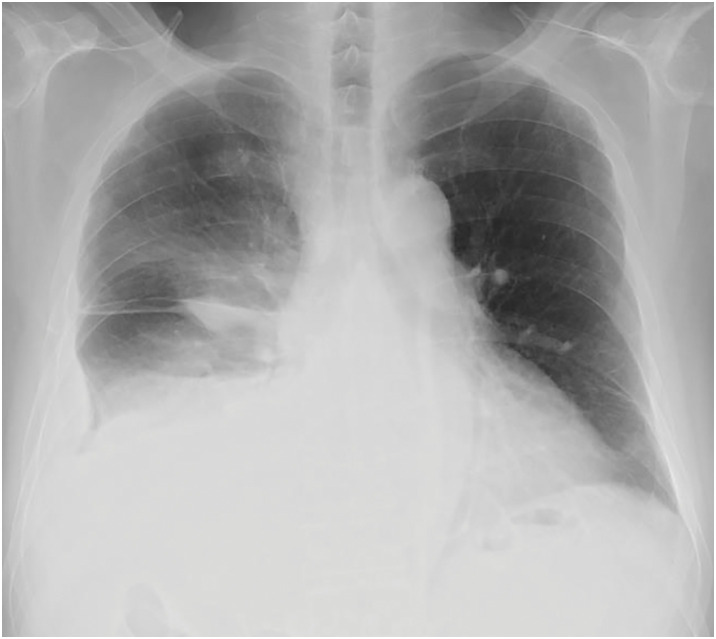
Chest X-ray image. Right hemidiaphragm elevation with right lower lobe atelectasis. Left hemithorax is normal.
